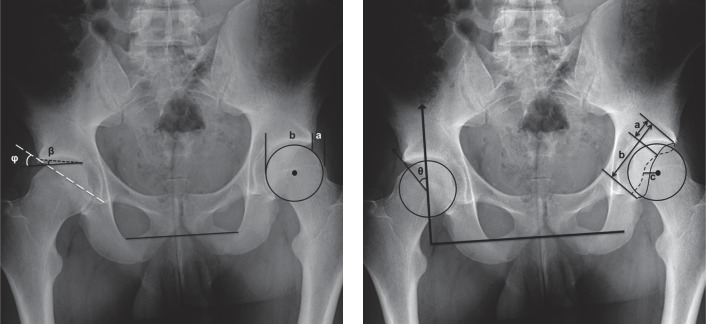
A. Right hip: The *acetabular index* is the angle (β) between the line parallel with pelvic tilt (solid black line which marks the horizontal reference) and the black dashed line from the medial sourcil (sclerotic radiographic density corresponding to the acetabular roof) to the lateral sourcil (where the sourcil meets the lateral acetabular rim). The *acetabular angle* (ϕ) is the angle made by the solid black line and the white dashed line from the acetabular teardrop to the lateral sourcil. Left hip: The *extrusion* index is the amount of femoral head uncovered by the acetabulum (distance a) divided by the diameter of the femoral head (distance a + distance b). B. Right hip: The *lateral center edge angle* (θ) is formed by a line passing through the center of the femoral head perpendicular to the inferior aspect of obturator foramina (thick black line) and a line from the center of the femoral head to the lateral aspect of the congruent sourcil (medial to calcified labra and up-sloping sourcil and even with the posterior wall). Left hip: *Crossover sign* is positive on the left, demonstrated by the anterior wall (solid) crossing the posterior wall (dashed). The *crossover*
